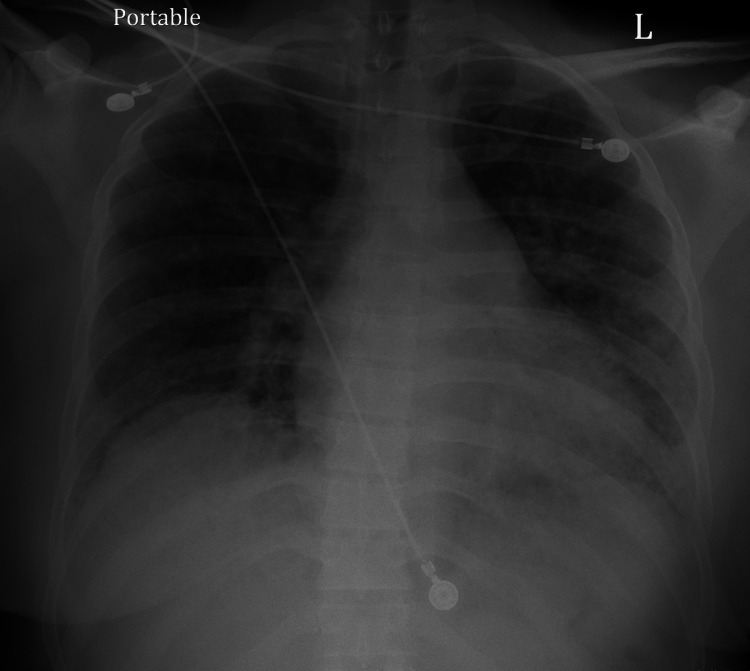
Anterior-posterior chest X-ray showing moderate pulmonary vascular congestion
